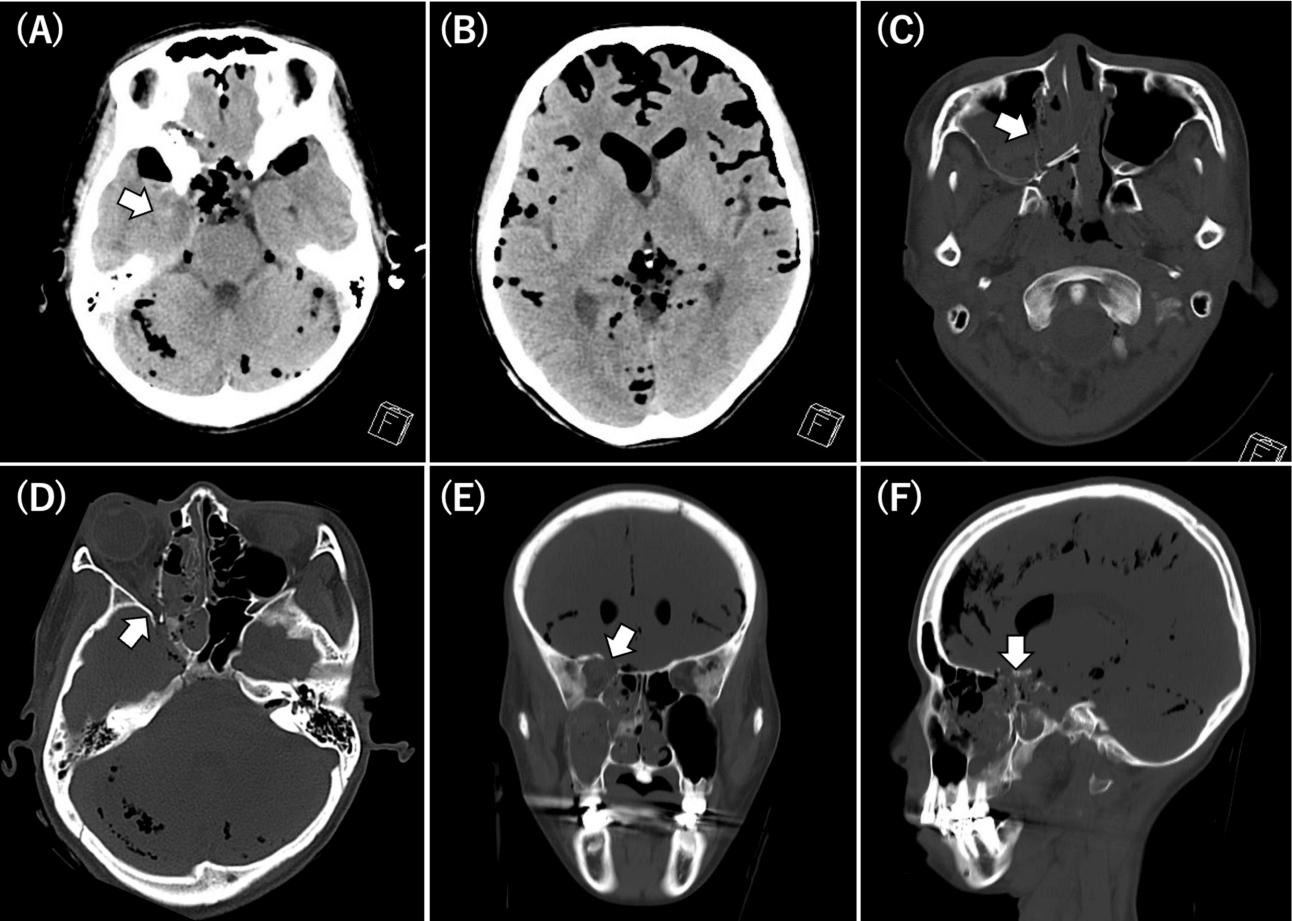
Head CT on admission. A: Right temporal lobe contusion (arrow). B: Extensive pneumocephalus along the cerebral sulci. C: Fractures from the maxillary sinus wall to the nasal septum(arrow). D: Optic canal fracture (arrow). E: Fractures of the orbital roof and sphenoid bone are observed in the coronal view (arrow). F: Fractures of the orbital roof and sphenoid bone are visualized in the sagittal view (arrow).
